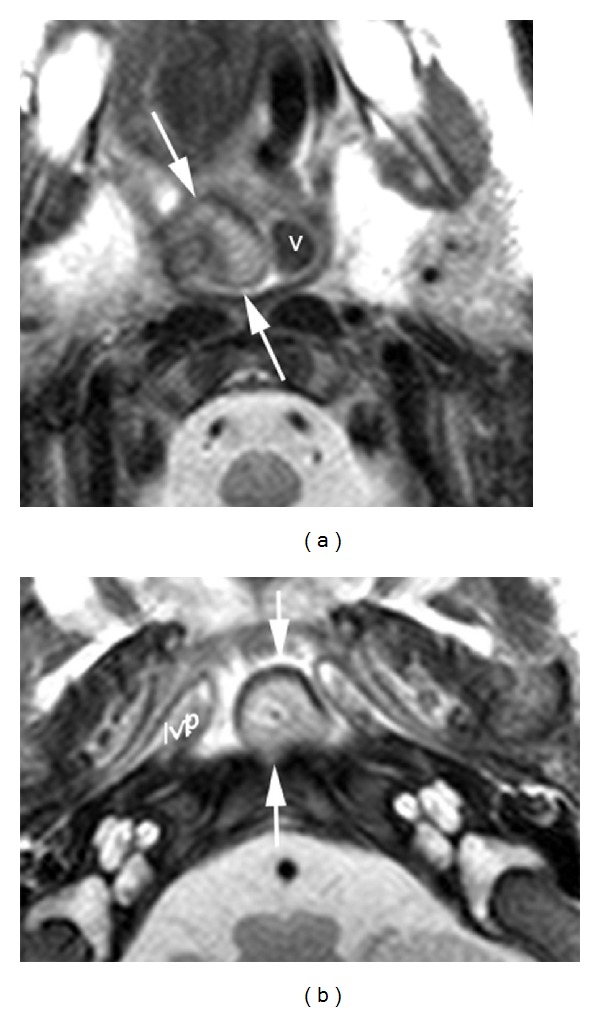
Axial T2-weighted image (a) at the level of the epiglottis displays that the lesion is arising from the right wall of the pharynx. The right vallecula is effaced. The left vallecula (v) is normal. Axial T2-weighted image (b) at the level of the nasopharynx shows the unattached margins of the mass (arrows). The black contour of the anterior wall of the lesion is due to chemical shift artifact, confirming the fat content of the lesion. Lvp: levator veli palatini muscle.
